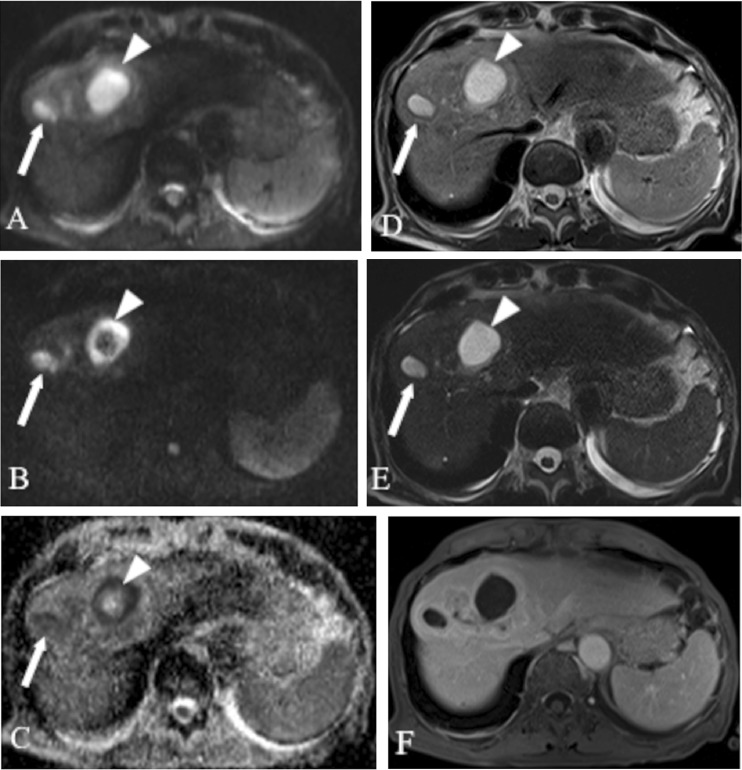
MR images obtained in an 83-year-old man with hepatic abscesses located in segment VIII. a
